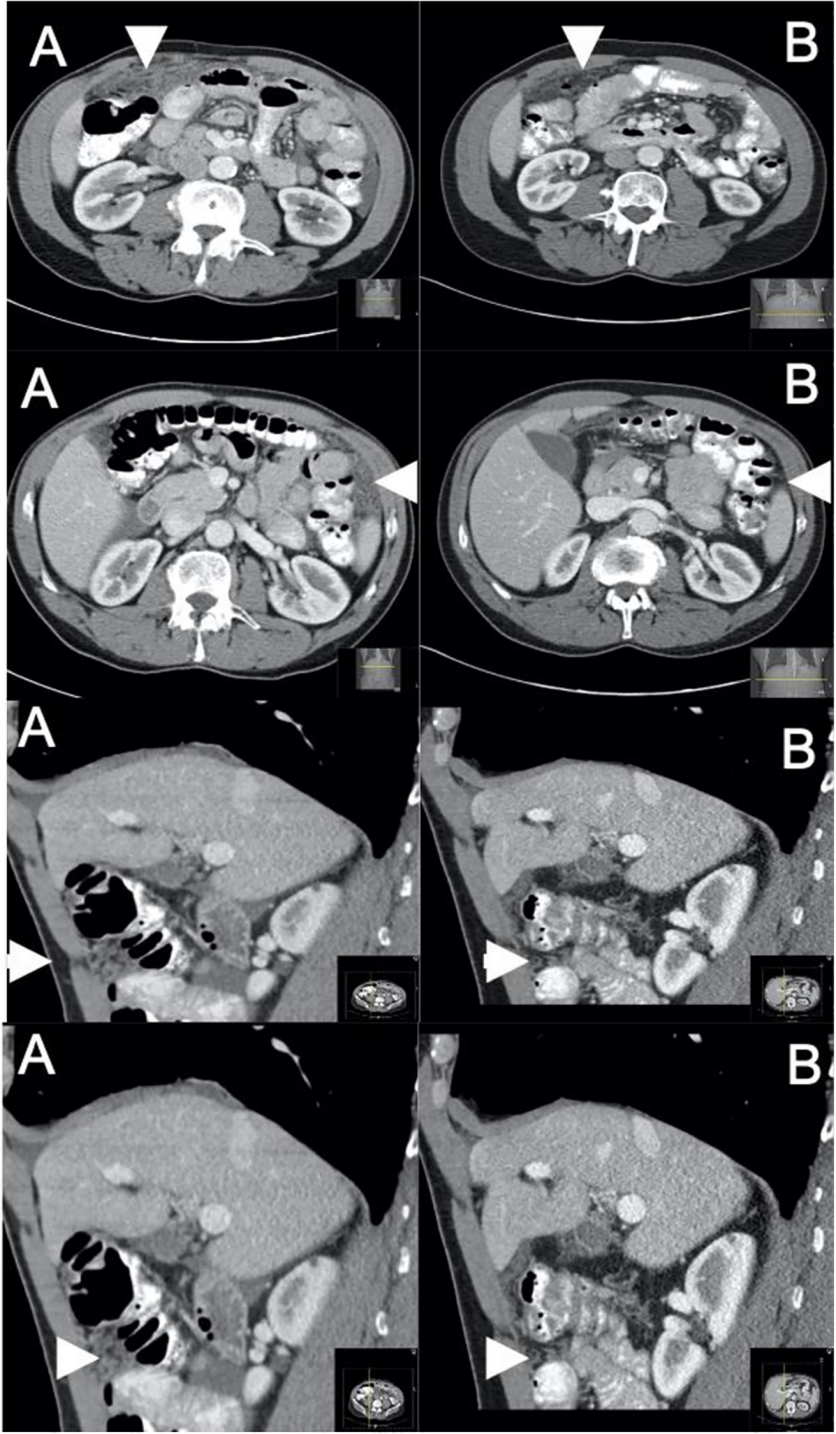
Partial response to neoadjuvant chemotherapy. Patient with DMPM with initial CT images prior to administration of systemic chemotherapy (A) and after six cycles of neoadjuvant carboplatin and pemetrexed (B), with marked partial response to therapy.
